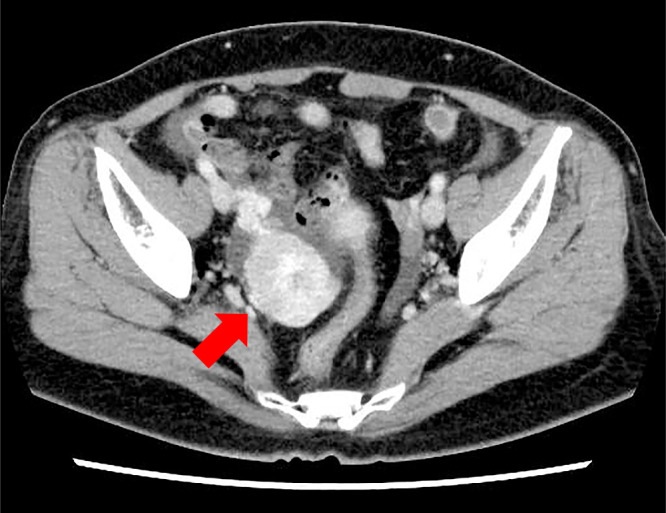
(Case 2) Contrast-enhanced computed tomography scan of the pelvic region (Case 2) A solid tumor of 48 mm in size with abundant blood flow was found in the right pelvis.
